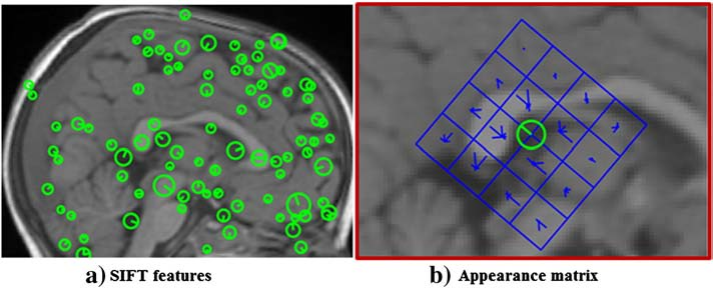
SIFT features. (a) SIFT features extracted from a 2D slice of a brain MR image. Every circle represents a feature. The center of a circle represents the location of the feature. The radius of the circle represents the scale of the feature. There is an appearance matrix associated with every SIFT feature. (b) An appearance matrix consists of a 4 × 4 squares. Every square contains an orientation histogram to represent the gradient orientations of the pixels within this square (Lowe, 1999). The length of the short lines within the squares represents the number of pixels with the corresponding gradient orientation.
